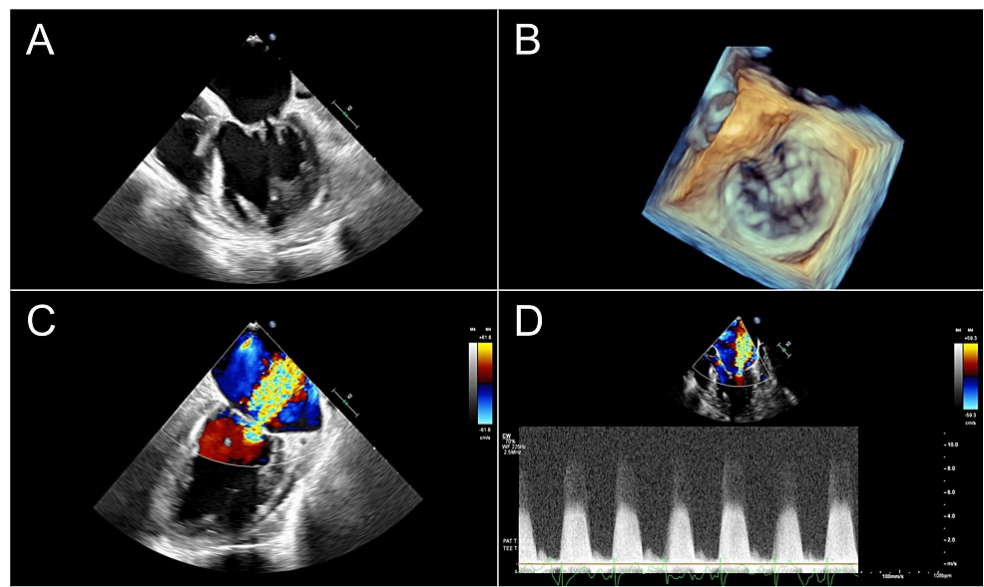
TEE imaging showing (A) mitral valve thickening with small free-moving vegetations, and (B) 3D rendering with posterior mitral valve leaflet restriction due to vegetations. (C) Doppler and (D) color Doppler studies demonstrating severe mitral regurgitation. TEE: transesophageal echocardiography
